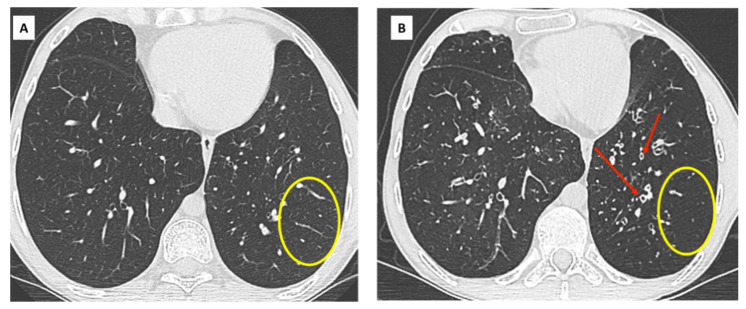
A 32-year-old man, bilateral LT in 2009 for cystic fibrosis. Diagnosis of BOS in 2011. (A) CT scan in January 2012 (FEV1 48%) shows no evidence of small or large airway disease; (B) CT scan in December 2017 (FEV1 18%, CLAD 4). Severe and diffuse bronchial wall thickening (red arrows), associated with mild bronchiectatic changes. Note the paucity of vascular markings in 2017 (yellow circles) compared to 2012 and subsequent hypoattenuation of lung parenchyma, related to small airway hypoventilation and fibrosis.
